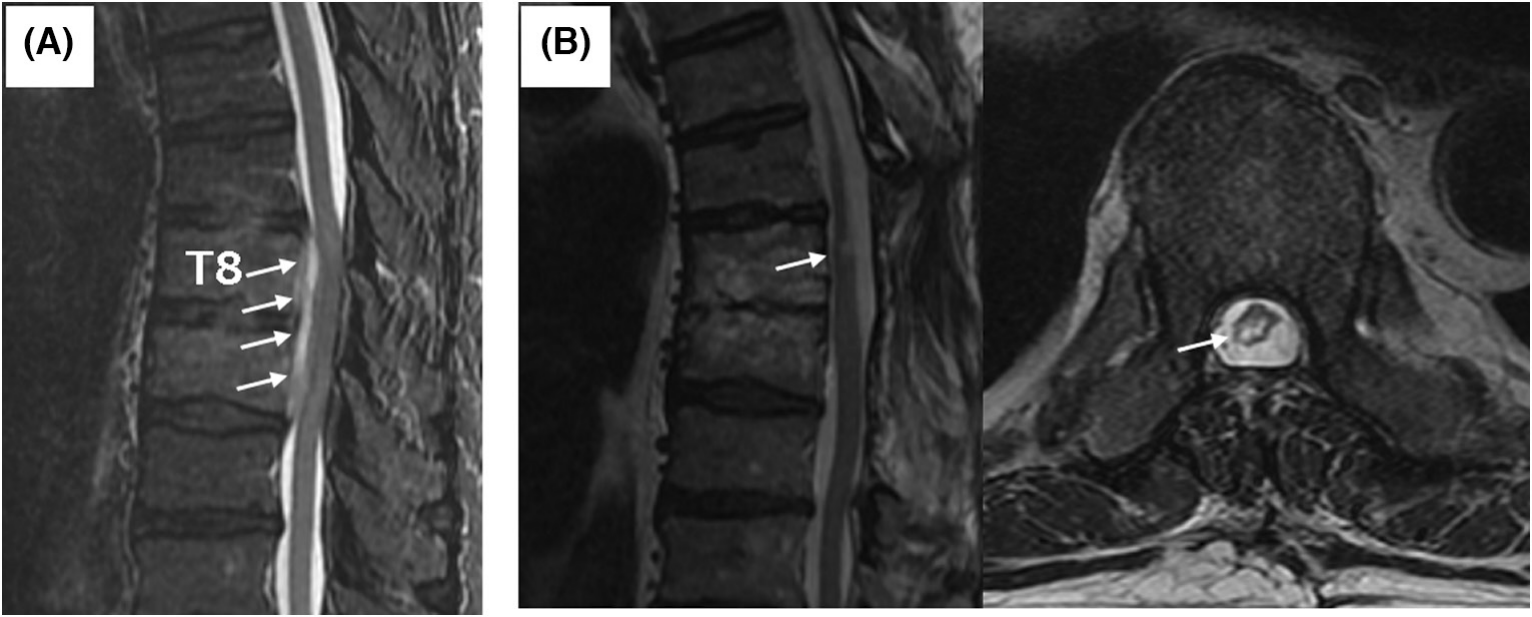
Magnetic resonance imaging of the thoracic spine before. (A) and 6 months after (B) treatment with pyogenic spondylitis. (A) Sagittal T2WI showing signal changes in the eighth and ninth thoracic vertebral body and epidural abscess (white arrows) compressing the spinal cord from the ventral side. (B) Epidural abscess and compression of the spinal cord were resolved, yet hyperintense signal change (white arrows) remains in sagittal and axial T2WI.
